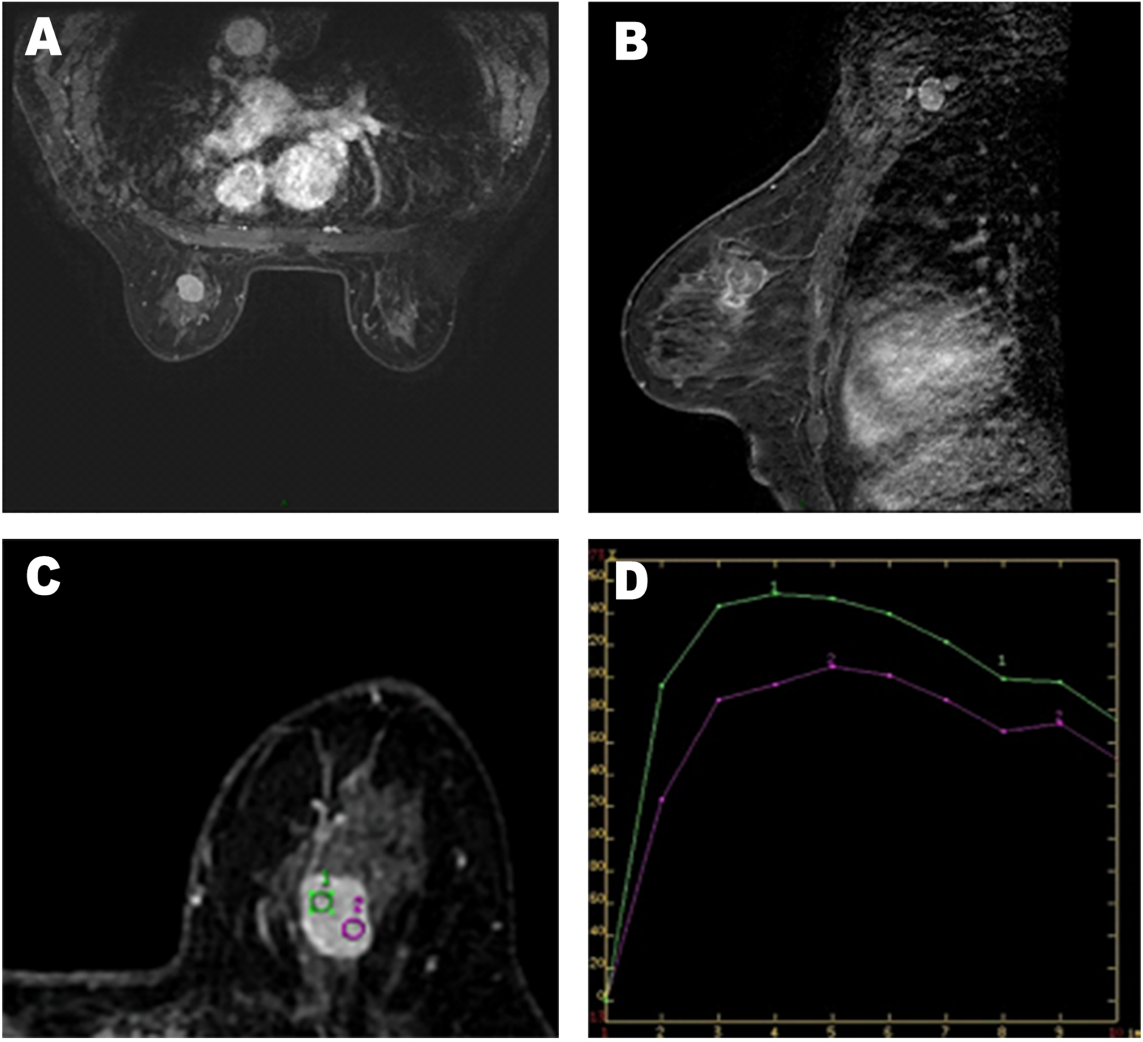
(A–C) Axial and sagittal contrast-enhanced MR image shows rapid and heterogeneous tumor enhancement. (D) Dynamic contrast-enhanced magnetic resonance imaging (MRI) demonstrated a time–signal intensity curve (TIC) with a rapid rise to a peak (after the administration of the contrast material), followed by a slow-out at the mass.
